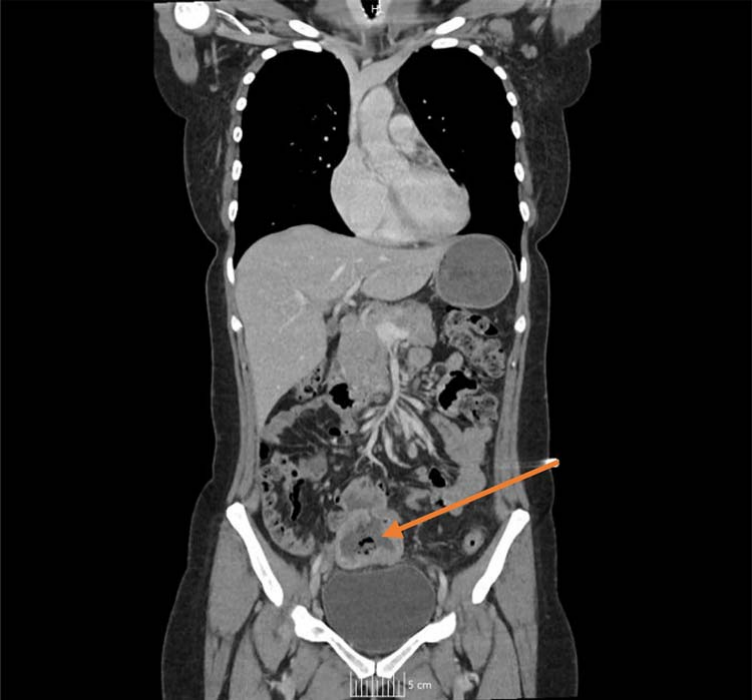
CT coronal image, with arrow showing the midline lower abdominal/pelvic thick walled fluid and gas-filled structure that measured 5 × 7 × 6 cm.
